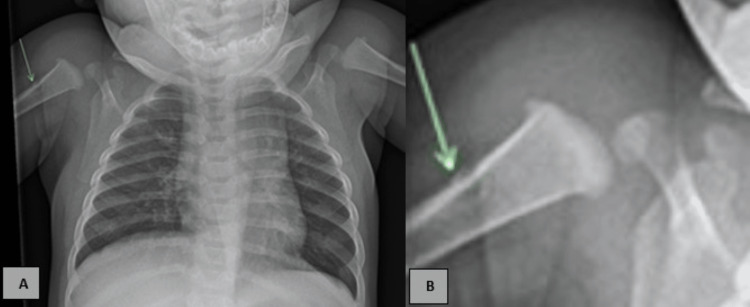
(A). Frontal chest radiograph demonstrates subtle cortical based lucency in the proximal right humeral metaphysis with irregular periosteal reaction (green arrow) with no other definite bony lesion seen. (B). Zoom down humerus image show ill-defined cortical irregularity.
